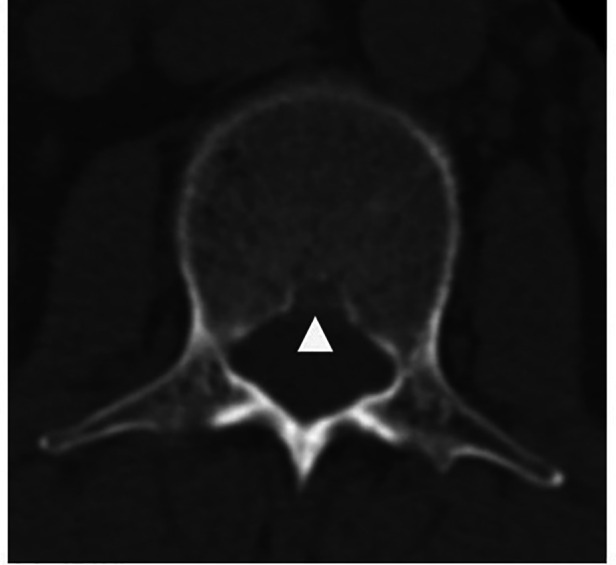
A wide BF is shown in CT scan (arrow head).
